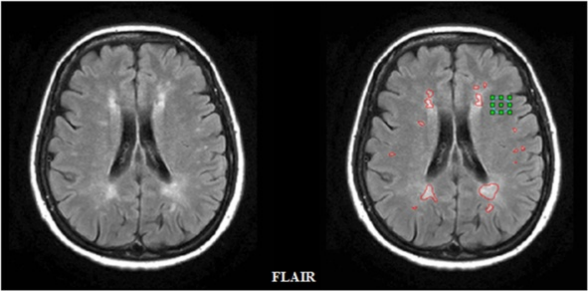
A descriptive example of semi-automated lesion segmentation process on fluid-attenuated inversion recovery (FLAIR) images. Segmented hyperintense lesions in red; area used for NAWM mean intensity estimation in green
